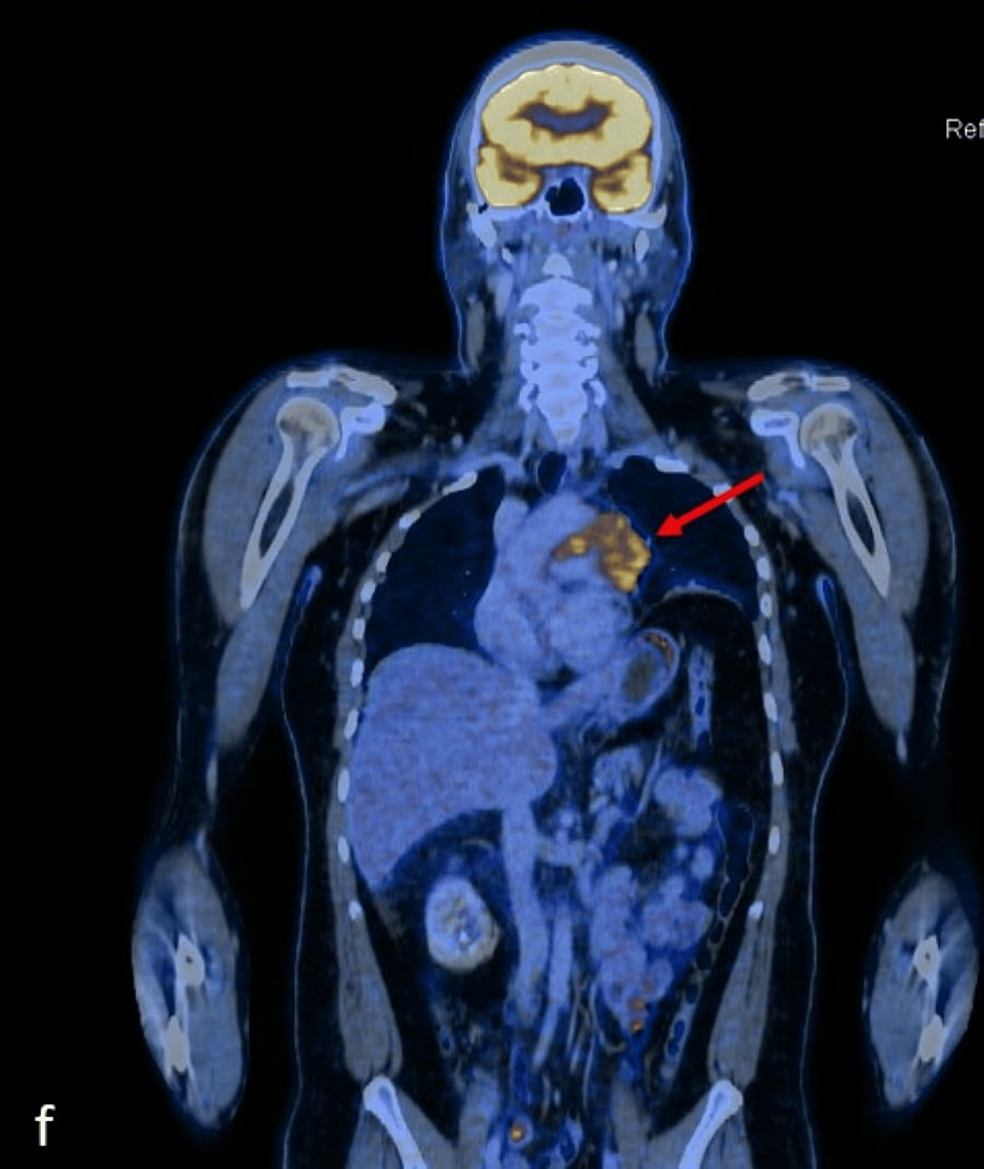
Coronal-fused positron emission tomography–computed tomography (PET CT) image of the chest and abdomen E and F show the elevated left dome of the diaphragm and thin subsegmental atelectasis of the lower lobe of the left lung, secondary to phrenic nerve invasion by the mediastinal mass.
